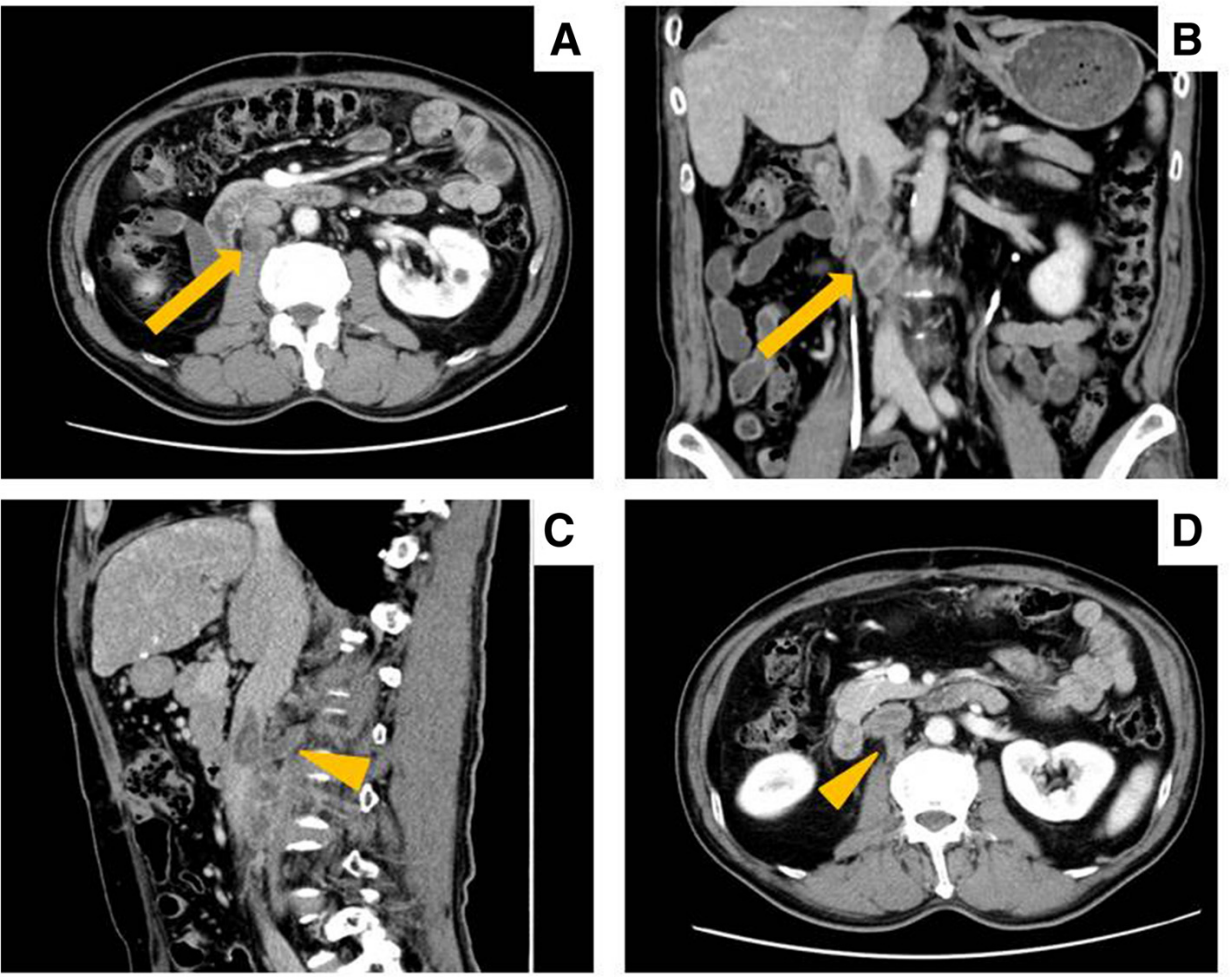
The paracaval lymph node metastases with an inferior vena cava tumor torombus. (A, B) Computed tomography revealed both enlarged lymph nodes (diameter, 3 cm; *arrow*), which were located behind the IVC, and (C, D) IVC tumor thrombus, which directly infiltrated through the lumbar vein (*arrowhead*).
